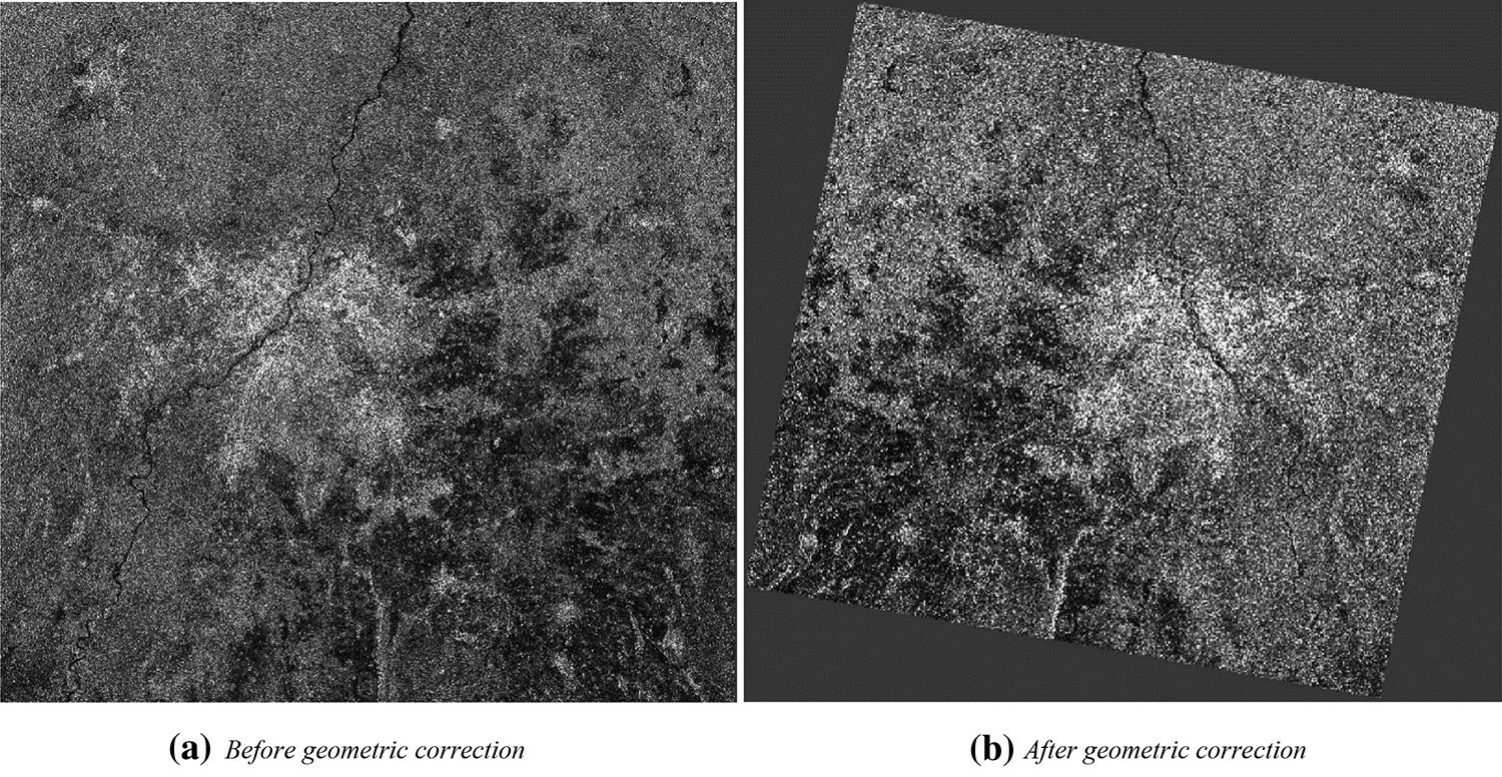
Effect of geometric correction/range Doppler terrain correction in VH&VV polarisation.
